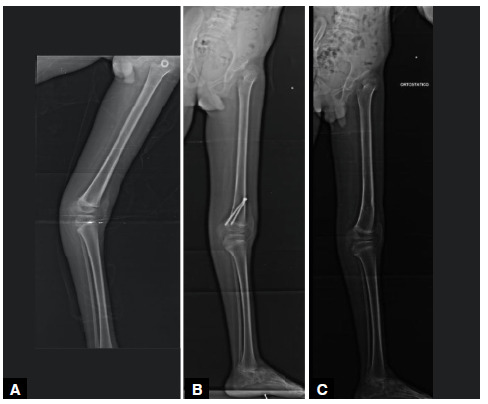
(A) Panoramic radiograph of the left lower limb in profile, a 10-year-old male patient, with a 33-degree knee recurvatum deformity due to arthrogryposis; (B) Panoramic radiograph of the left lower limb in profile, 1 year and 5 months after surgical treatment with posterior epiphysiodesis of the distal femur, showing correction of the deformity; (C) Panoramic radiograph in profile, 1 year and 1 month after the removal of the epiphysiodesis, with maintenance of the correction.
